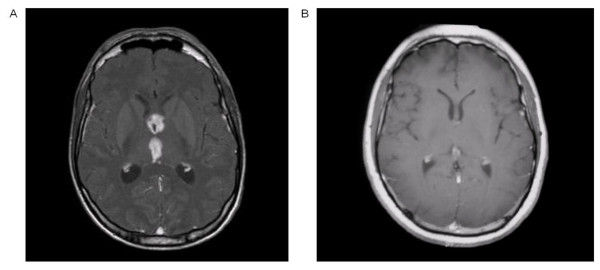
Complete response to vinblastine chemotherapy. Third ventricle lesion. Axial T1-weighted magnetic resonance imaging (MRI) with gadolinium before vinblastine treatment (A) and 6 months after treatment initiation, showing a complete response (B).
